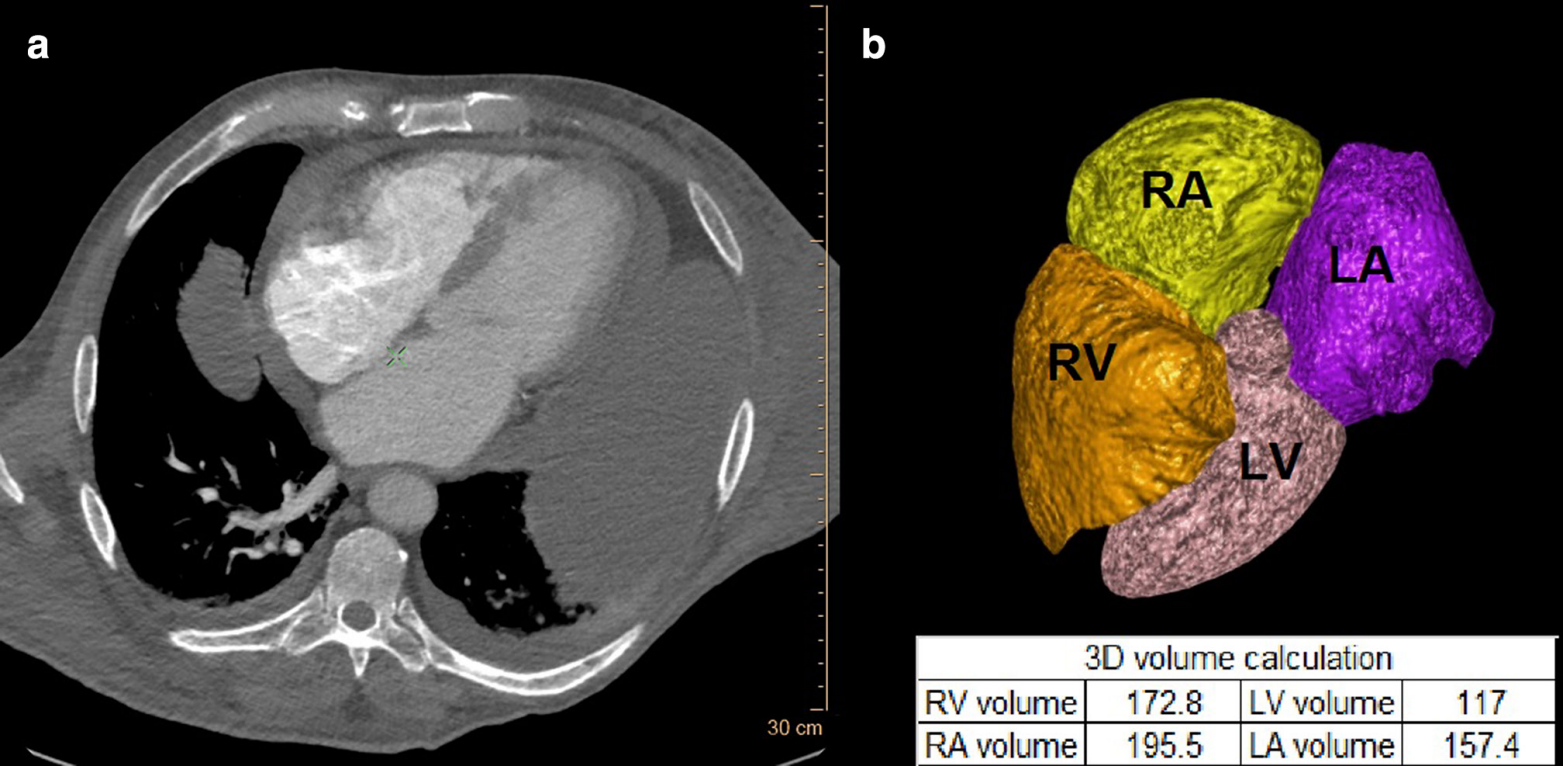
Representative case. Volumetric analysis of the CT pulmonary angiography (CTPA) of a 77-year-old male who presented to the emergency department with shortness of breath and clinical signs of right heart failure. CTPA ruled out pulmonary embolism, but there was a large pericardial effusion. His volumetric analysis was LV volume 117 ml, LA volume 157.4 ml, RV volume 172.8 ml, and RA volume 195.5. Echocardiography showed a moderate pericardial effusion with no signs of hemodynamic significance. He was provisionally diagnosed as having combined diastolic heart failure with COPD and appropriate treatment led to significant improvement.
